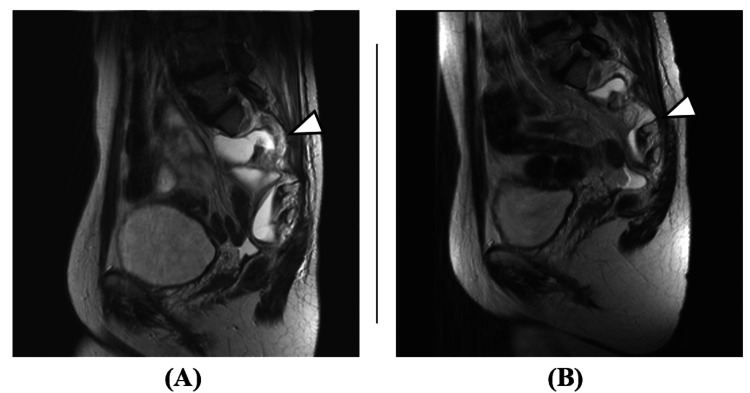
Postoperative pelvic MRI (T2 sagittal) (A) One week after surgery, (B) Two months after surgery The cyst wall (arrowheads) regressed over time.
